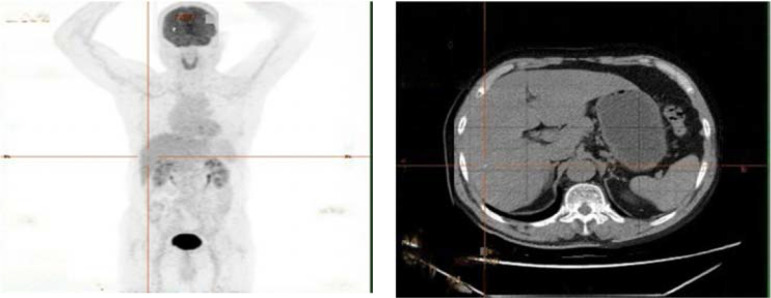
2022-09–24 PET-CT demonstrated complete resolution of previously documented hepatic lesions and nodal involvement, with no evidence of FDG-avid disease.
